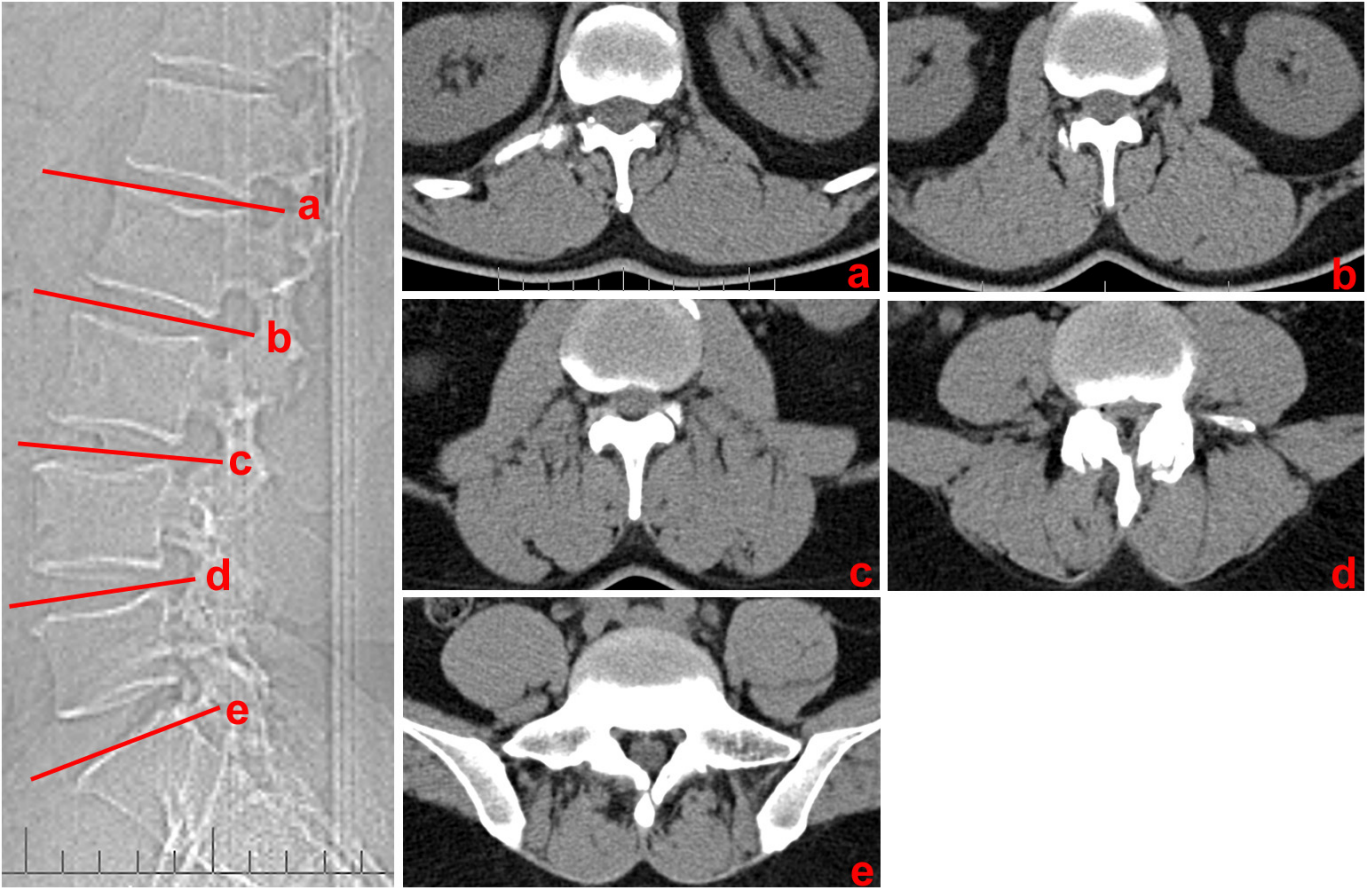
Transverse CT images at different disc level. (a) L1-L2 intervertebral disc level; (b) L2-L3 intervertebral disc level; (c) L3-L4 intervertebral disc level; (d) L4-L5 intervertebral disc level; (d) L5-S1 intervertebral disc level
